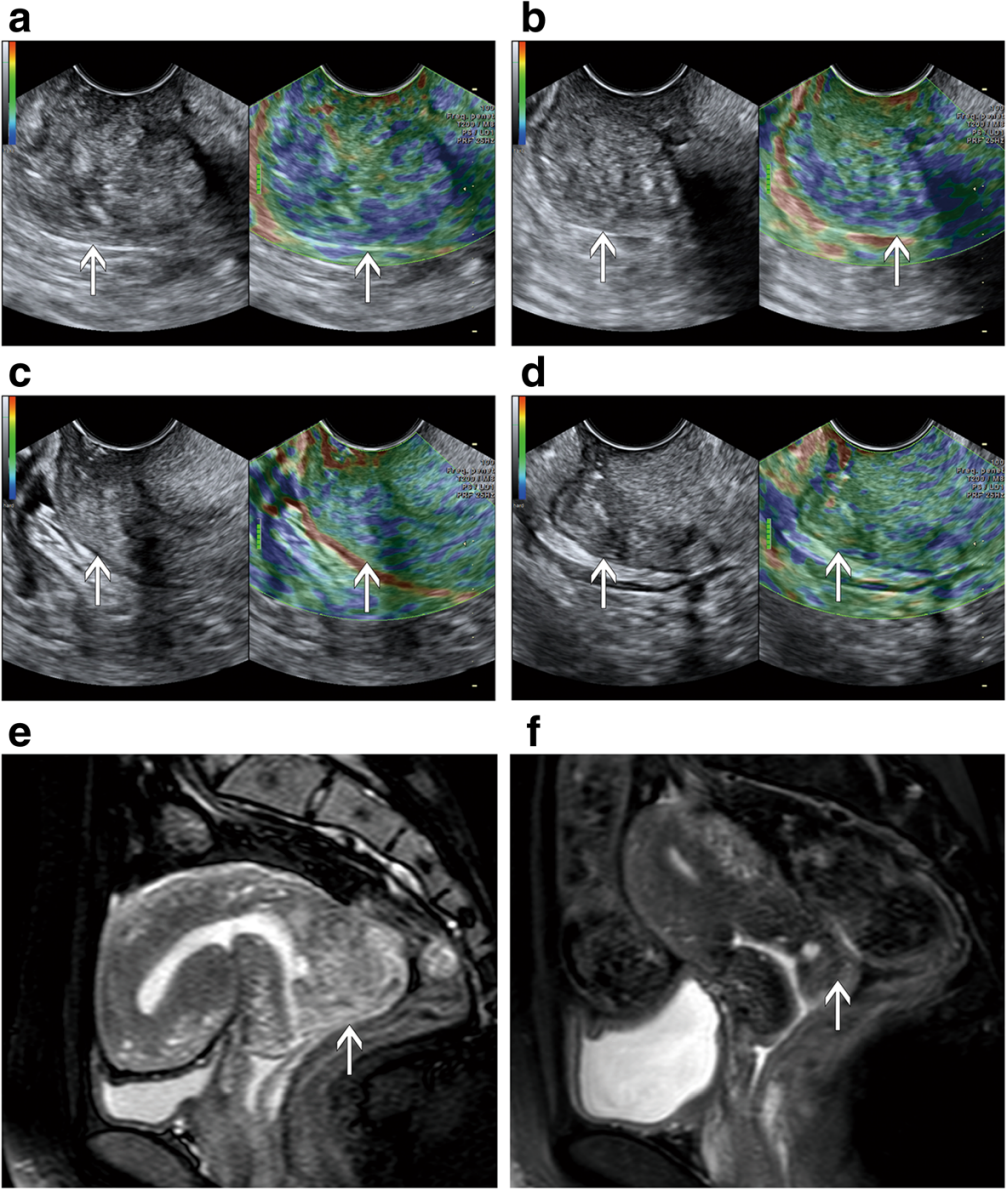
A patient with advanced cervical cancer (FIGO stage IIA) experienced partial response to CCRT. B-mode and elastography images show a consecutive decrease in strain ratio in cervical cancer (arrow): 4.16 before therapy a 3.56 at week 1 during CCRT b 3.07 at week 2 during CCRT c and 2.73 post CCRT d Corresponding axial T2-weighted images exhibited a decrease in the maximal diameter of tumor (arrows): 3.3 cm at pre-therapy e and 1.2 cm post therapy f
